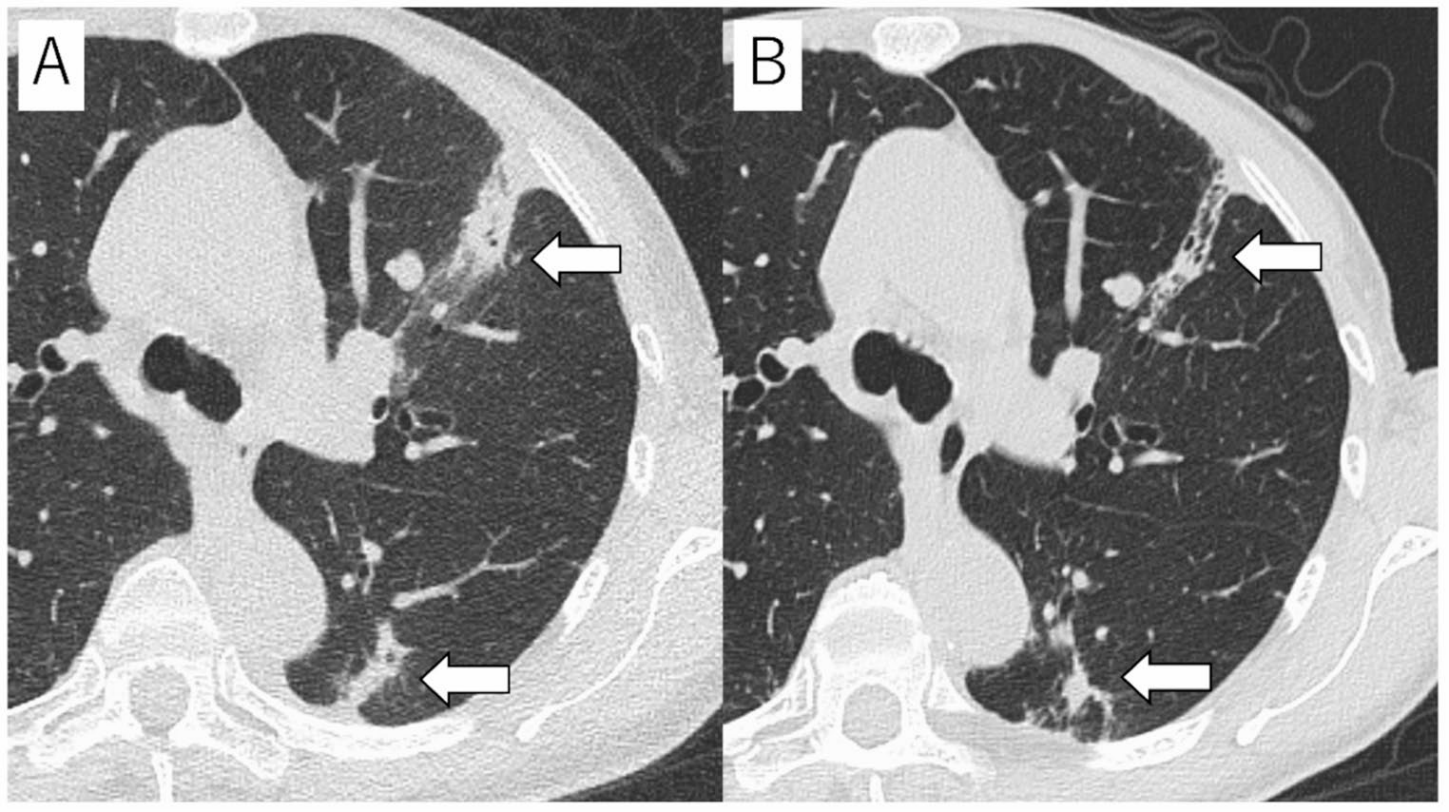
High-resolution CT (HRCT) images of Trastuzumab Deruxtecan (T-DXd)-related pneumonitis in a 76-year-old woman. The patient had multiple lung metastases from luminal HER2 type breast cancer, and was receiving T-DXd. HRCT image shows multiple consolidations in the left upper and lower lobes of the lung (arrows) (A). Drug-associated interstitial lung disease with an organizing pneumonia (OP) pattern due to T-DXd was suspected. Since the patient was asymptomatic, the severity is classified as grade 1 according to the Common Terminology Criteria for Adverse Events (CTCAE), and treatment was temporarily discontinued. A follow-up HRCT image obtained two months later shows decreased consolidation (arrows) (B), and there were no further changes, so administration was resumed at a reduced dose
